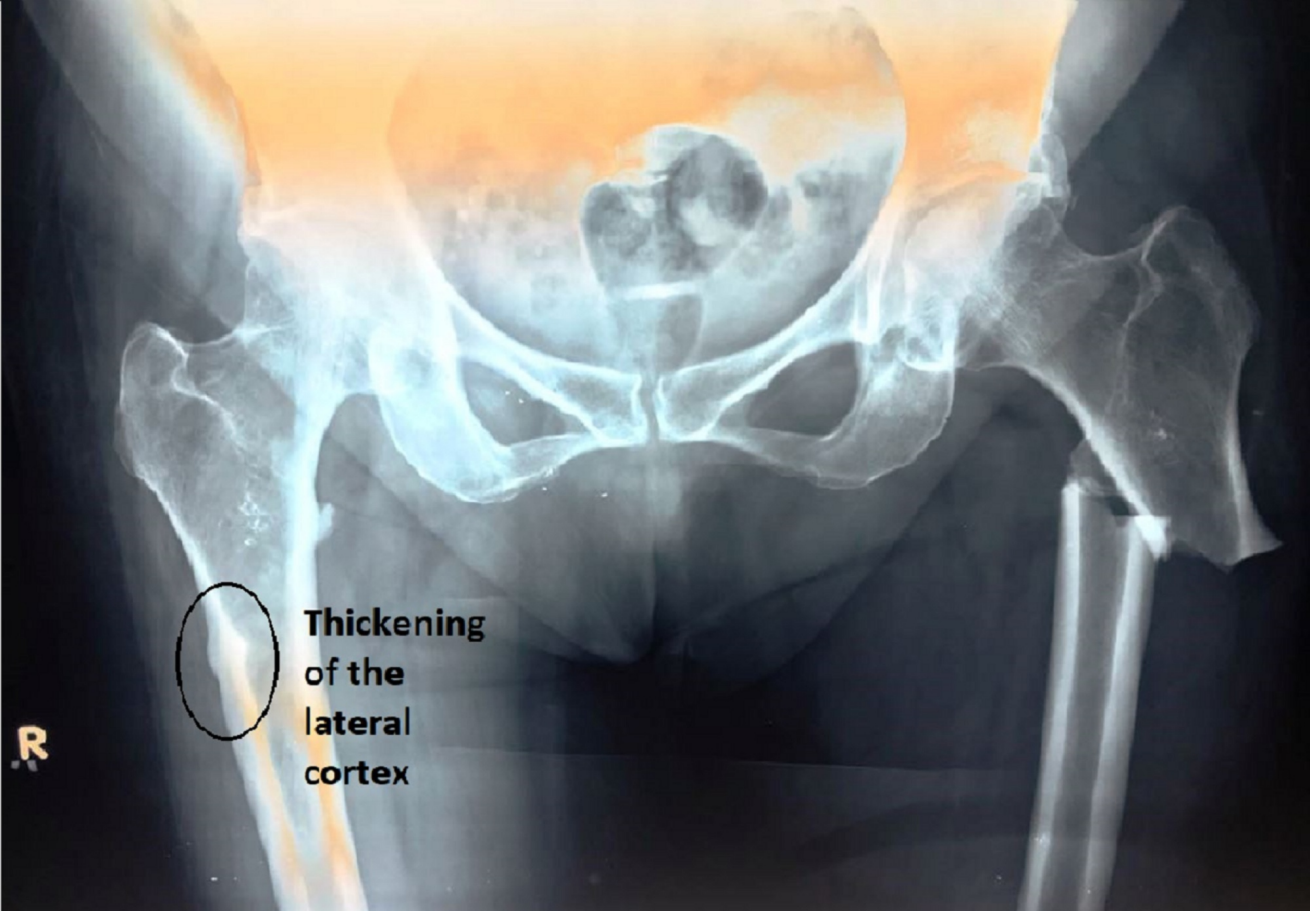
X-ray pelvis with hips antero-posterior view showing subtrochanteric fracture of the left femur with localized thickening of the lateral cortex of right femur subtrochanteric region.
